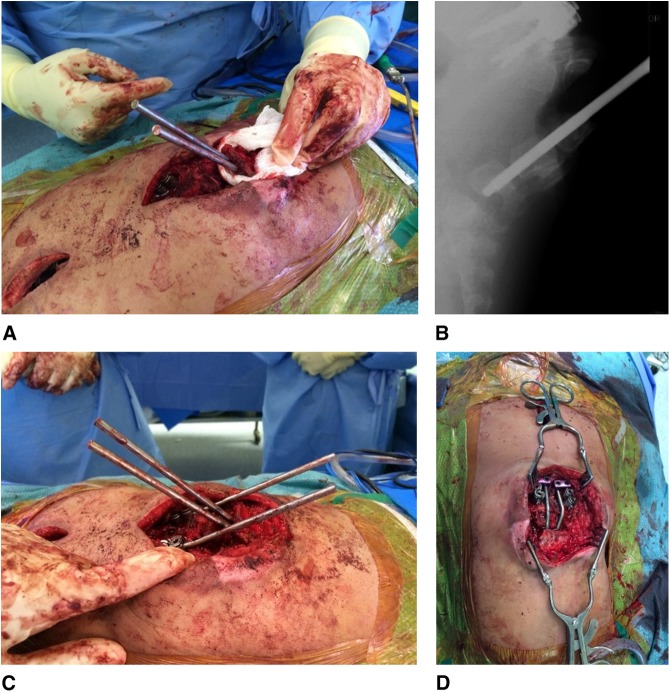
A, Titanium rods were inserted through the superior end plate of the L3 vertebral body through to the inferior end plate of L5. B, Lateral intraoperative radiograph showing insertion of spinal rods through the vertebral bodies of L3, L4, and L5. C, The distal rod ends that formed the proximal growing rod construct were left long at this point to allow for connection to the distal rod construct. The proximal and distal rod constructs were then brought together in a drawbridge maneuver to correct the kyphosis and connect the proximal and distal instrumentation. The proximal upper thoracic incision for insertion of the T2/T3 pedicle screws is visible at the left-hand side of the image. D, The connection between the distal rod construct—bent accordingly to facilitate connection to the domino connectors attached to the proximal growing rod construct. The pedicle screws at T9/T10 are visible at the proximal extent of the incision.
